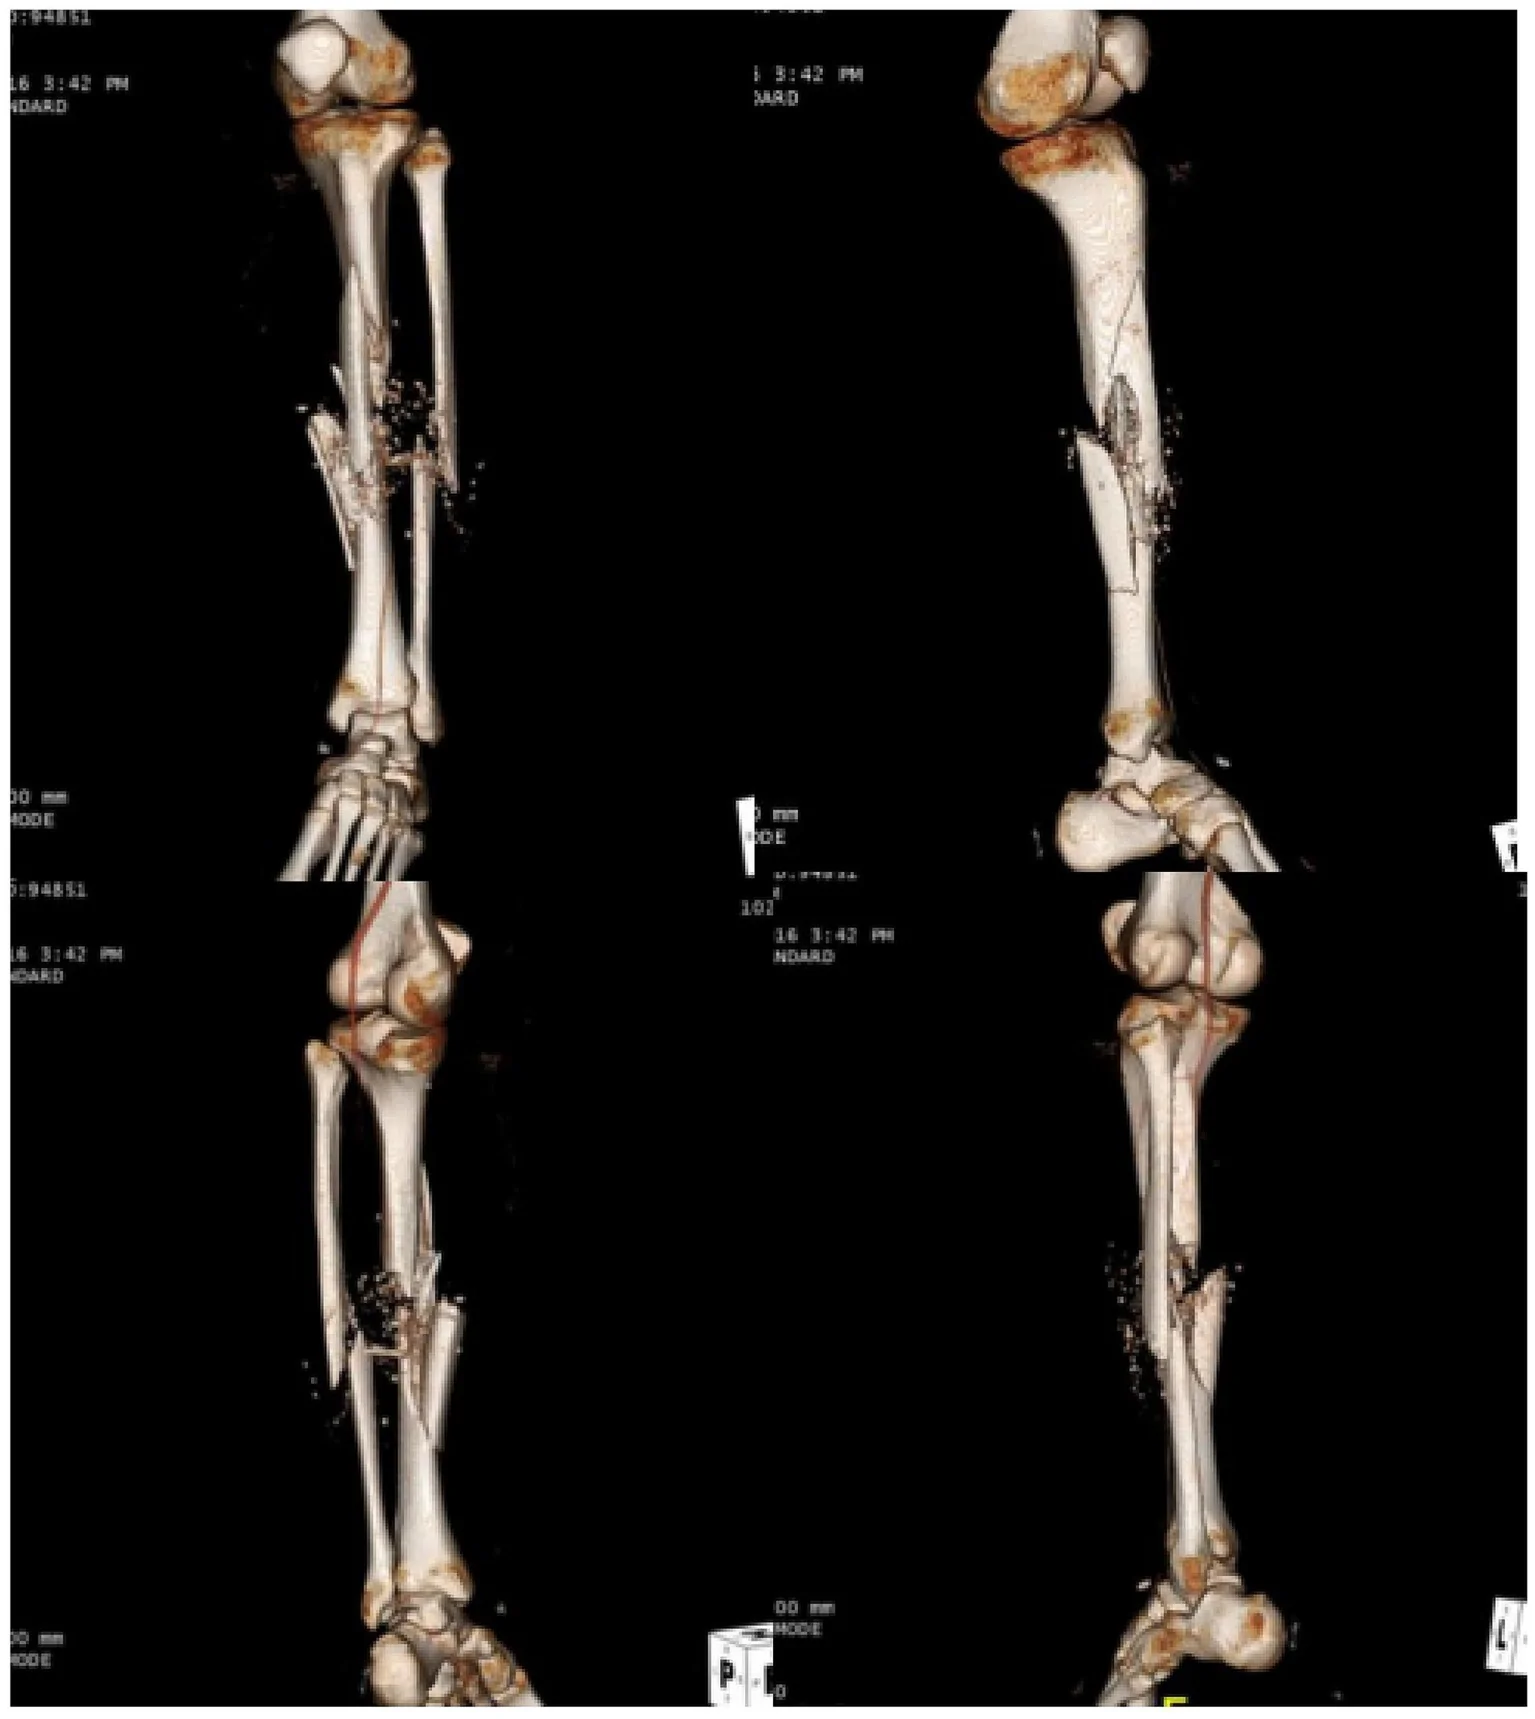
Post-injury three-dimensional CT imaging revealed a comminuted fracture of the tibia and fibula, with a significant amount of bullet fragments visible at the fracture site.
